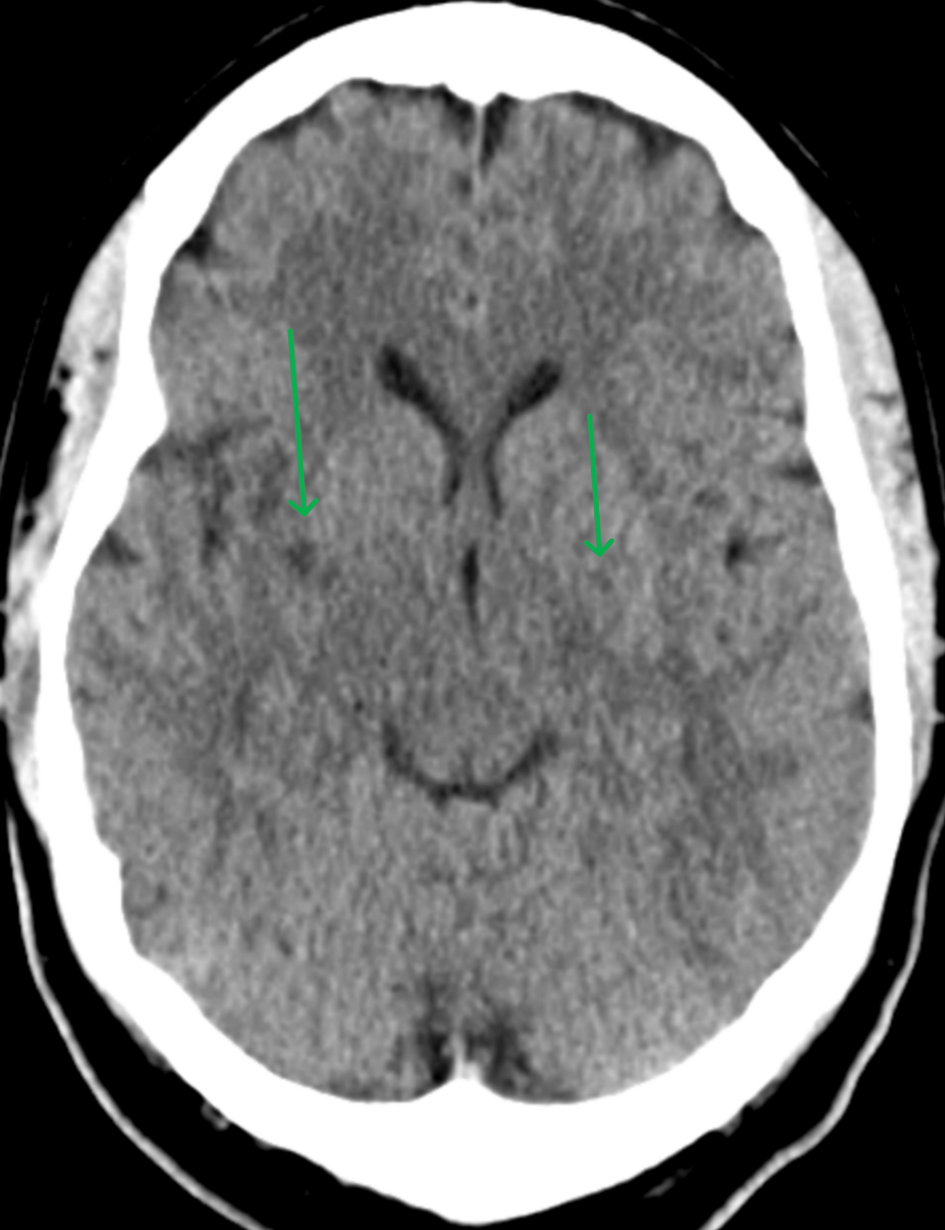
Cranial CT scan 1 Axial cranial CT scan showing lacunar infarcts in the bilateral basal ganglia and external capsule. No evidence of intracranial hemorrhage. The age of infarcts is indeterminate.
